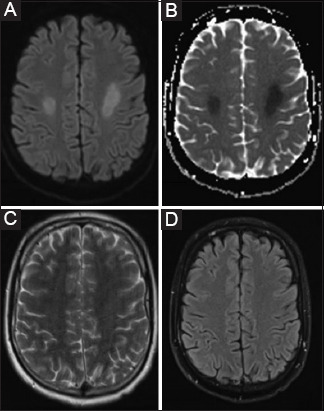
Magnetic resonance imaging cerebrum, 24 hours after the development of neurological deficits. (A) Diffusion-weighted imaging. (B) Apparent diffusion coefficient images: Bilateral diffusion restriction corona radiata/semi-oval center, (C) T2. (D) Fluid-attenuated inversion recovery: No abnormalities.
